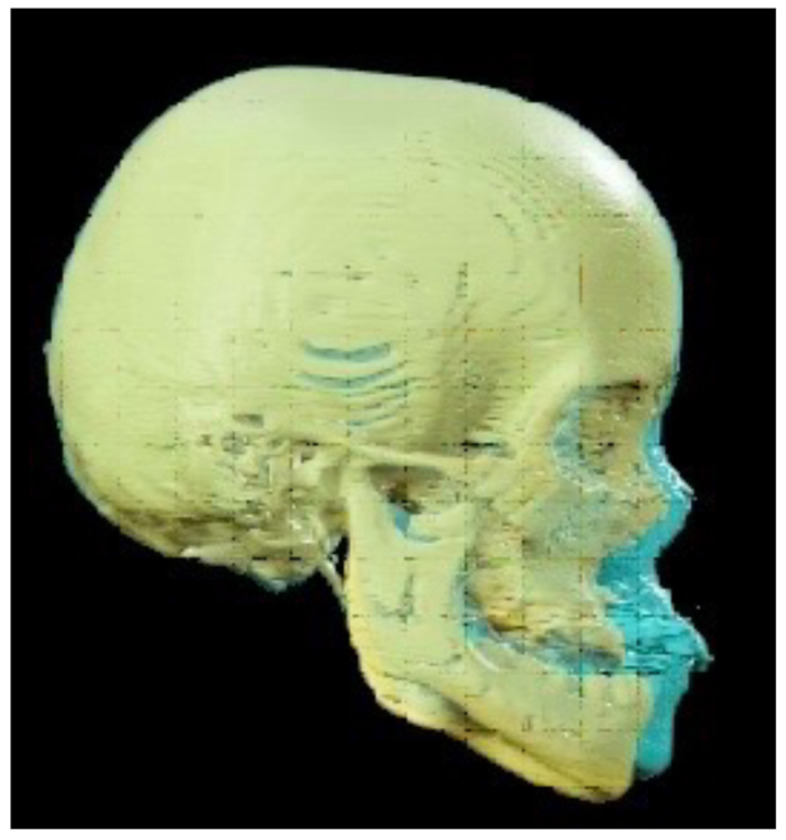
Superimposition of 3D models obtained from CT scans: the skull reconstruction before treatment is highlighted in yellow, while the one after treatment is depicted in blue.
